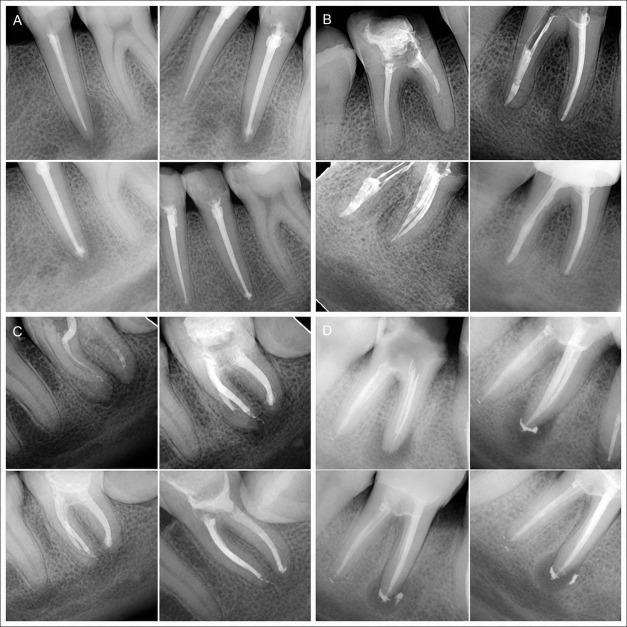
Postoperative and follow-up radiographs from representative cases with apical periodontitis. (A) Mandibular first premolar, 2-year follow-up, ranked as healed. (B) Mandibular first molar, 2-year follow-up, ranked as healed. (C) Mandibular second molar, 2-year follow-up. Apical extrusion of a separated instrument is present. The tooth met the predefined radiographic and clinical criteria for healing (PAI ≤ 2, asymptomatic), with no radiographic progression observed across follow-up; (D) Maxillary first premolar, 1-year follow-up, ranked as diseased.
